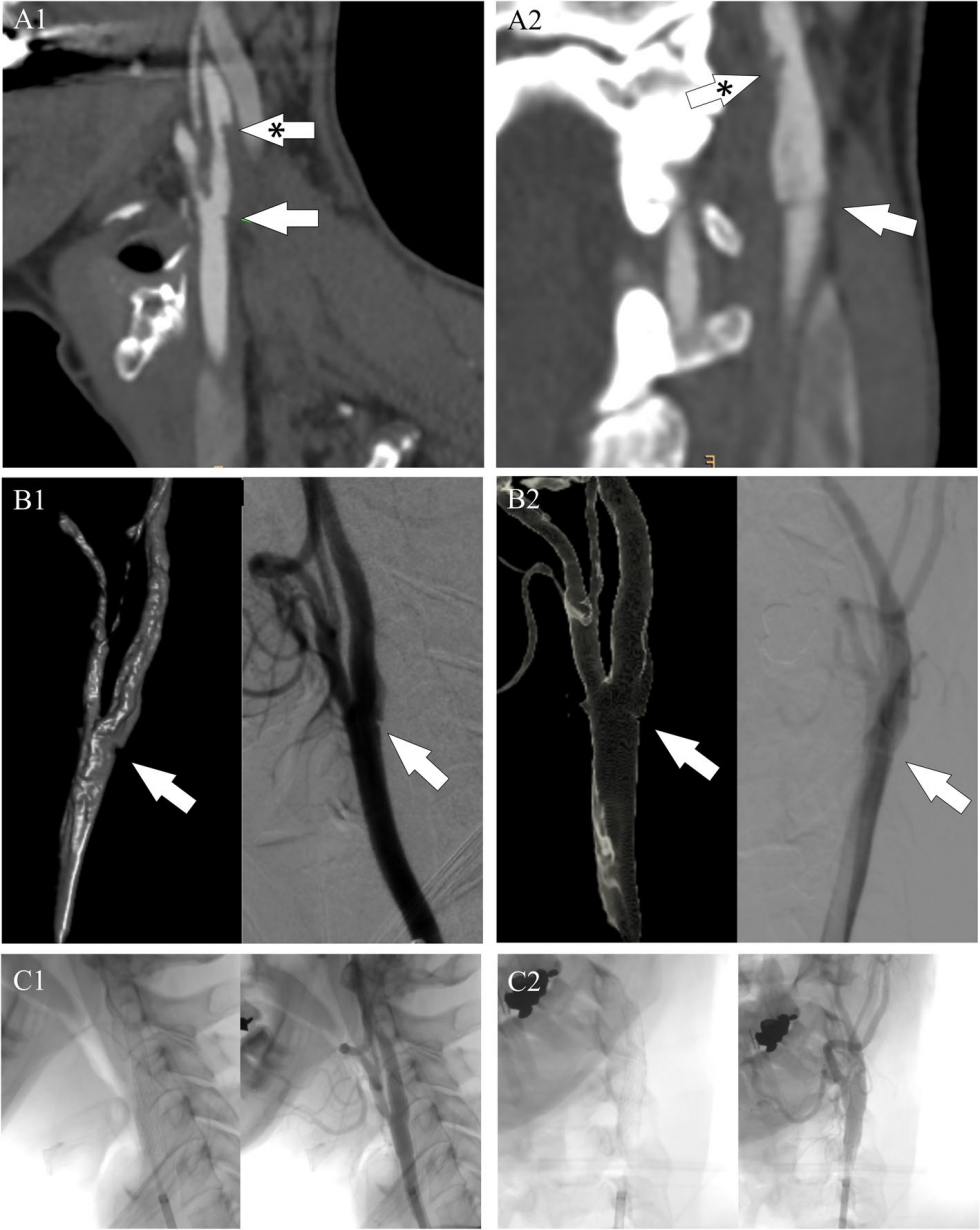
Sagittal CT (A1) and coronal 3D rotational (A2) angiography showed a linear filling defect located in the posterior internal carotid artery bulb consistent with a CaW (white arrow) and a vertically elongated hypodensity partially attached to the below carotid wall consistent with CFFT (asterisk-marked arrow). Intraoperative DSA and 3D reconstruction confirmed the presence of a CaW (arrow) on sagittal (B1) and coronal (B2) projections. Post-operative DSA showing appropriate stent placement and flow restoration on sagittal (C1) and coronal (C2) projections. CFFT: carotid free-floating thrombus. CaW: carotid web. DSA: digital subtraction angiography
